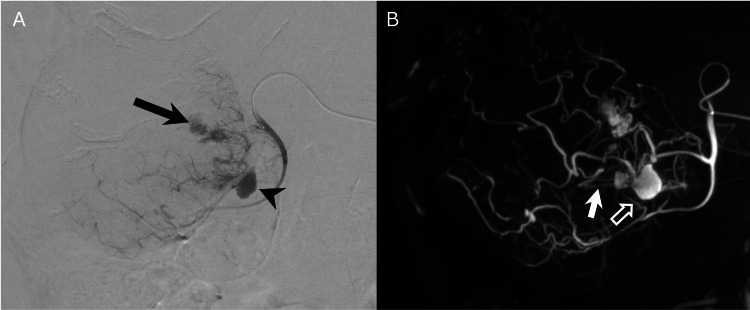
Angiogram and cone-beam computed tomography angiography from a cystic artery (A) An angiogram from a cystic artery demonstrates extravasation within the tumor (black arrow) and a pseudoaneurysm (black arrowhead). (B) Cone-beam computed tomography angiography demonstrates the branches from both the superficial (white hollow arrow) and deep (white arrow) cystic arteries, supplying the pseudoaneurysm.
